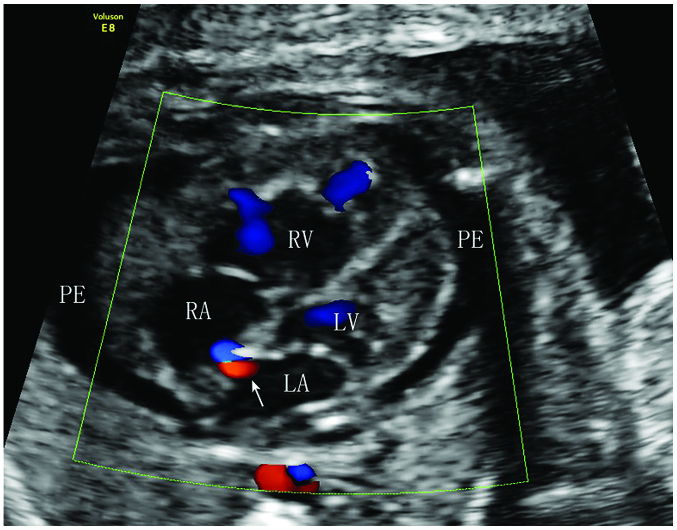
Color Doppler imaging indicating an extremely narrow foramen ovale flow (indicated by the white arrow). PE, pericardial effusion; RA, right atrium; RV, right ventricle; LV, left ventricle; LA, left atrium.
